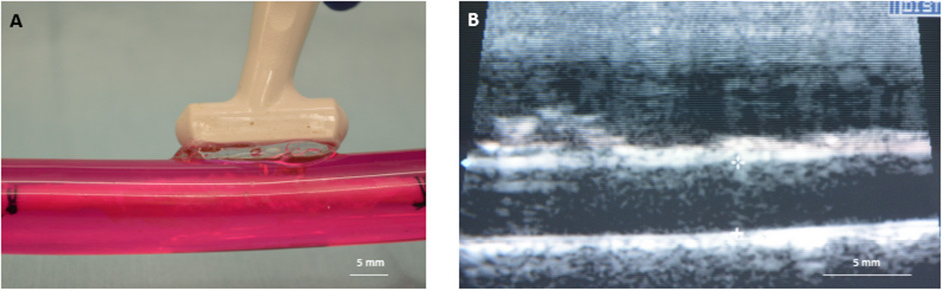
Diameter measurement of explanted arteries. (A) An ultrasound probe can be directly placed on the outer sleeve of the bioreactor housing to measure the diameter. (B) Ultrasound image showing the inner diameter of the explanted artery.
